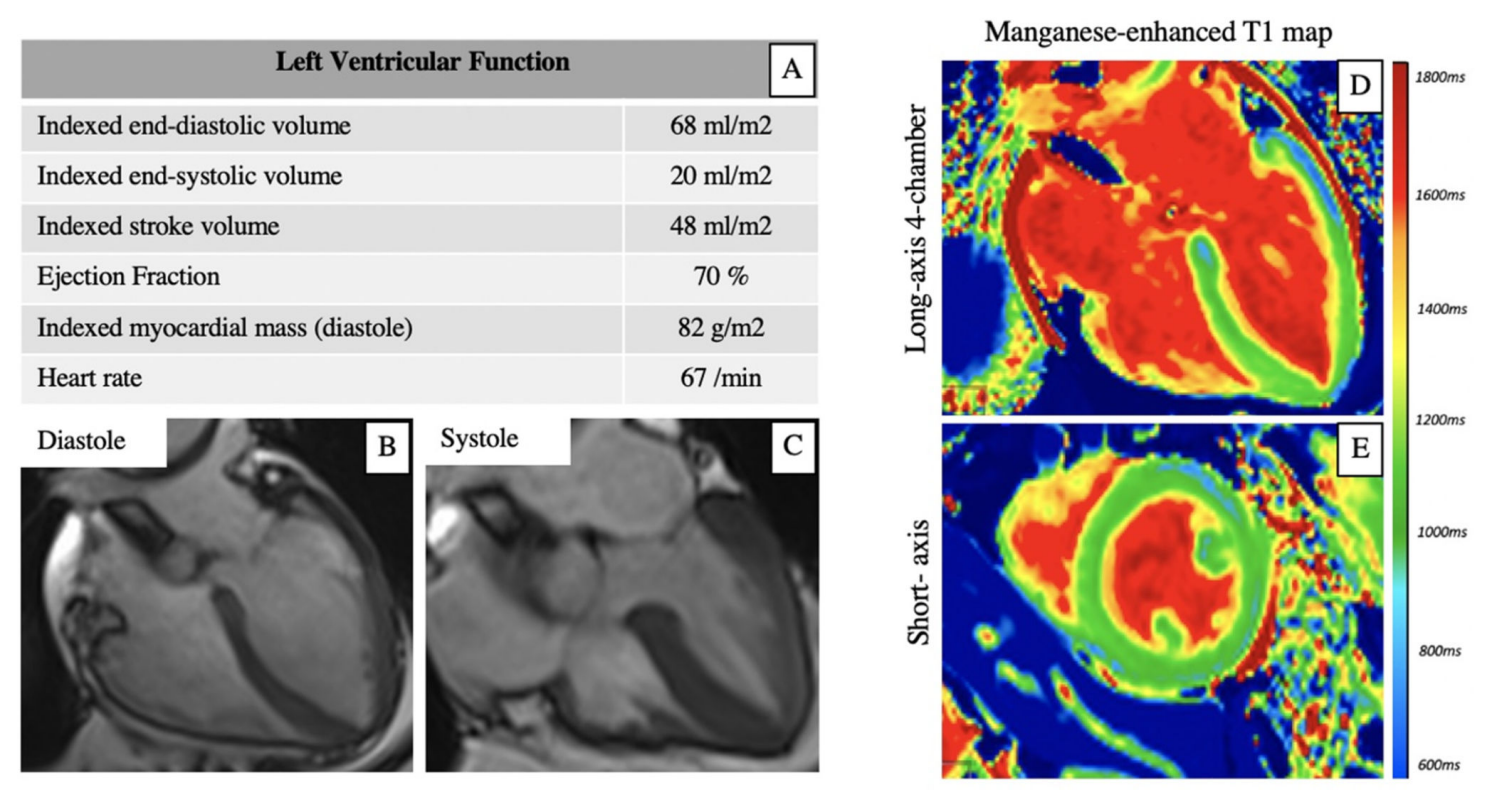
Manganese-enhanced Magnetic Resonance Imaging in Takotsubo Syndrome Resolution of left ventricular systolic dysfunction (A) and apical ballooning (B and C) in a patient with takotsubo syndrome scanned 18 days after symptom onset. Long-axis (D) and short-axis (E) manganese-enhanced T1 map demonstrating typical apical pattern of takotsubo syndrome with abnormal myocardial manganese uptake (green) in mid-ventricular and apical segments with normal uptake (blue) in basal segments despite apparent restoration of normal cardiac function.
